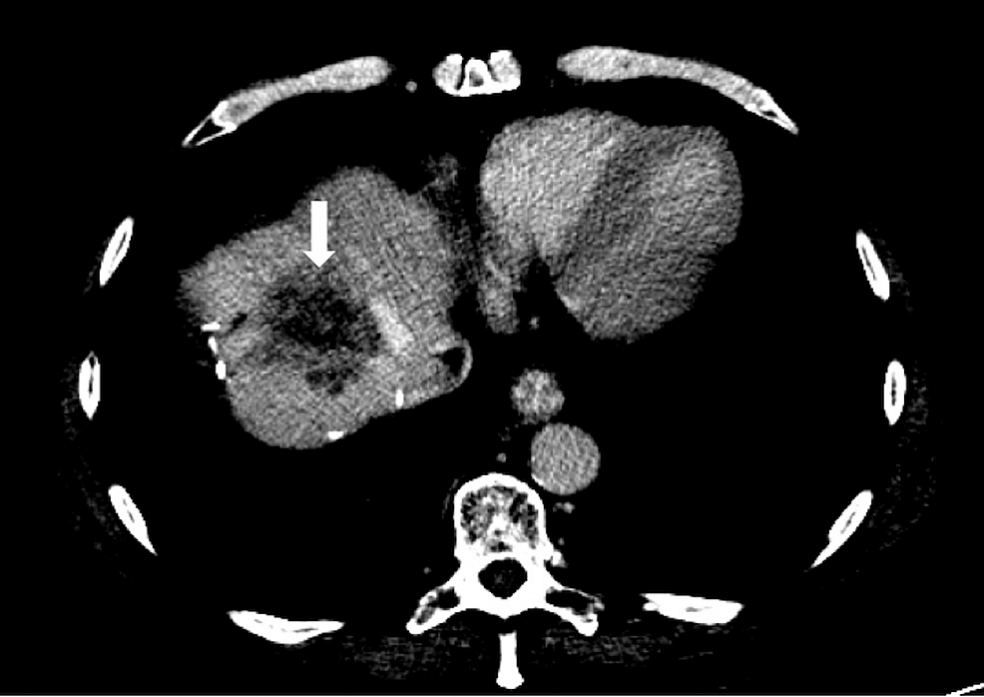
Axial CT abdomen in the portovenous phase in liver window demonstrating the segment 4a tumor (white arrow).
